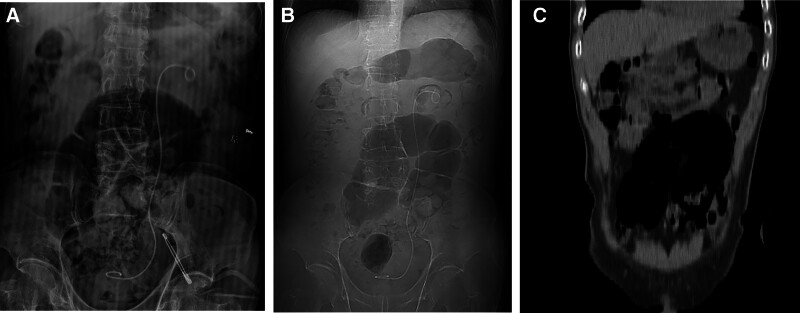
(A) An abdominal plain radiograph demonstrated an inverted U-shaped distended sigmoid colon with a maximum diameter of approximately 7 cm, exhibiting the classic “coffee bean sign.” (B) Scout view from a CT scan. (C) Coronal plane reconstruction and 3D reconstruction, revealing “coffee bean sign.” CT = computed tomography.
